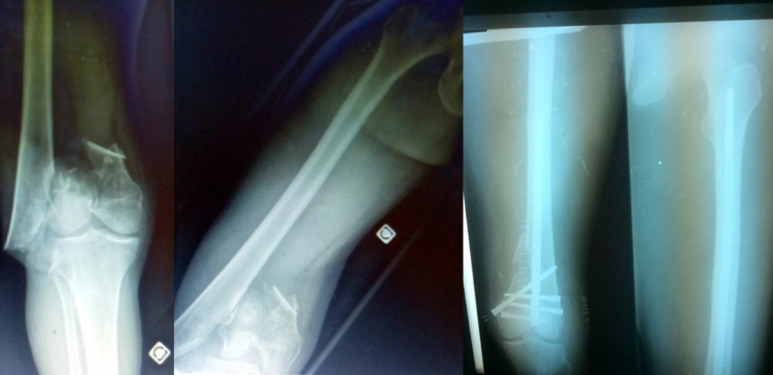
fracture sus et inter condylienne du fémur distal et radiographies post opératoires après implantation d´un *Fin Nail* (Bujumbura - Burundi)
